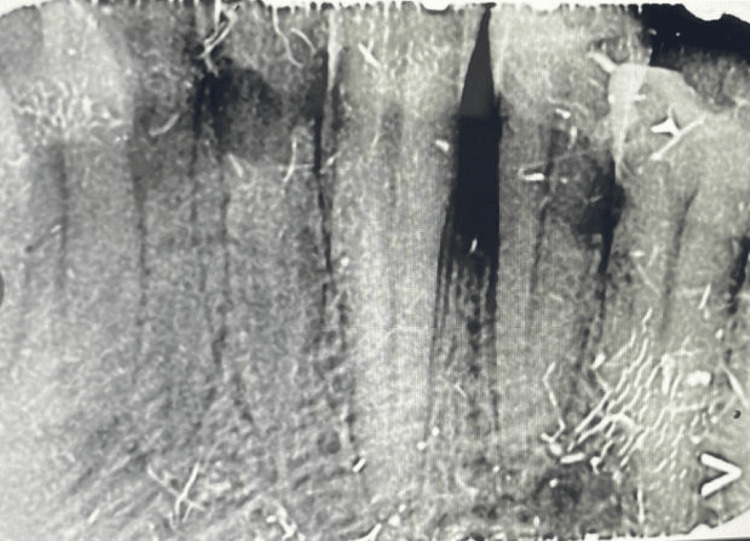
Intraoral periapical radiograph showing interdental bone loss in the 32-33 region
